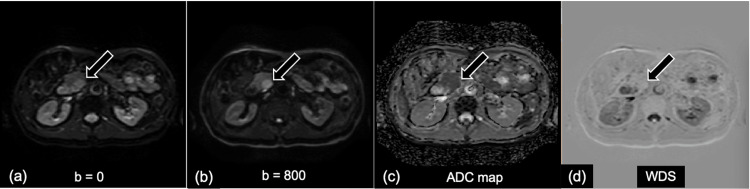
A representative case of a man in his 60s with pancreatic ductal adenocarcinoma (PDAC) in the pancreatic head. (a) diffusion-weighted image (DWI) b value = 0 s/mm^2^, (b) DWI b values = 800 s/mm^2^, (c) apparent diffusion coefficient (ADC) map, (d) weighted diffusion subtraction (WDS) image. (a, b) On the DWI (b = 0, 800 s/mm^2^), an irregularly shaped mass located in the head of the pancreas shows high signal intensity compared with the normal pancreas parenchyma. (d) The WDS image shows the mass with peripheral slightly high signal intensity and central low intensity.
